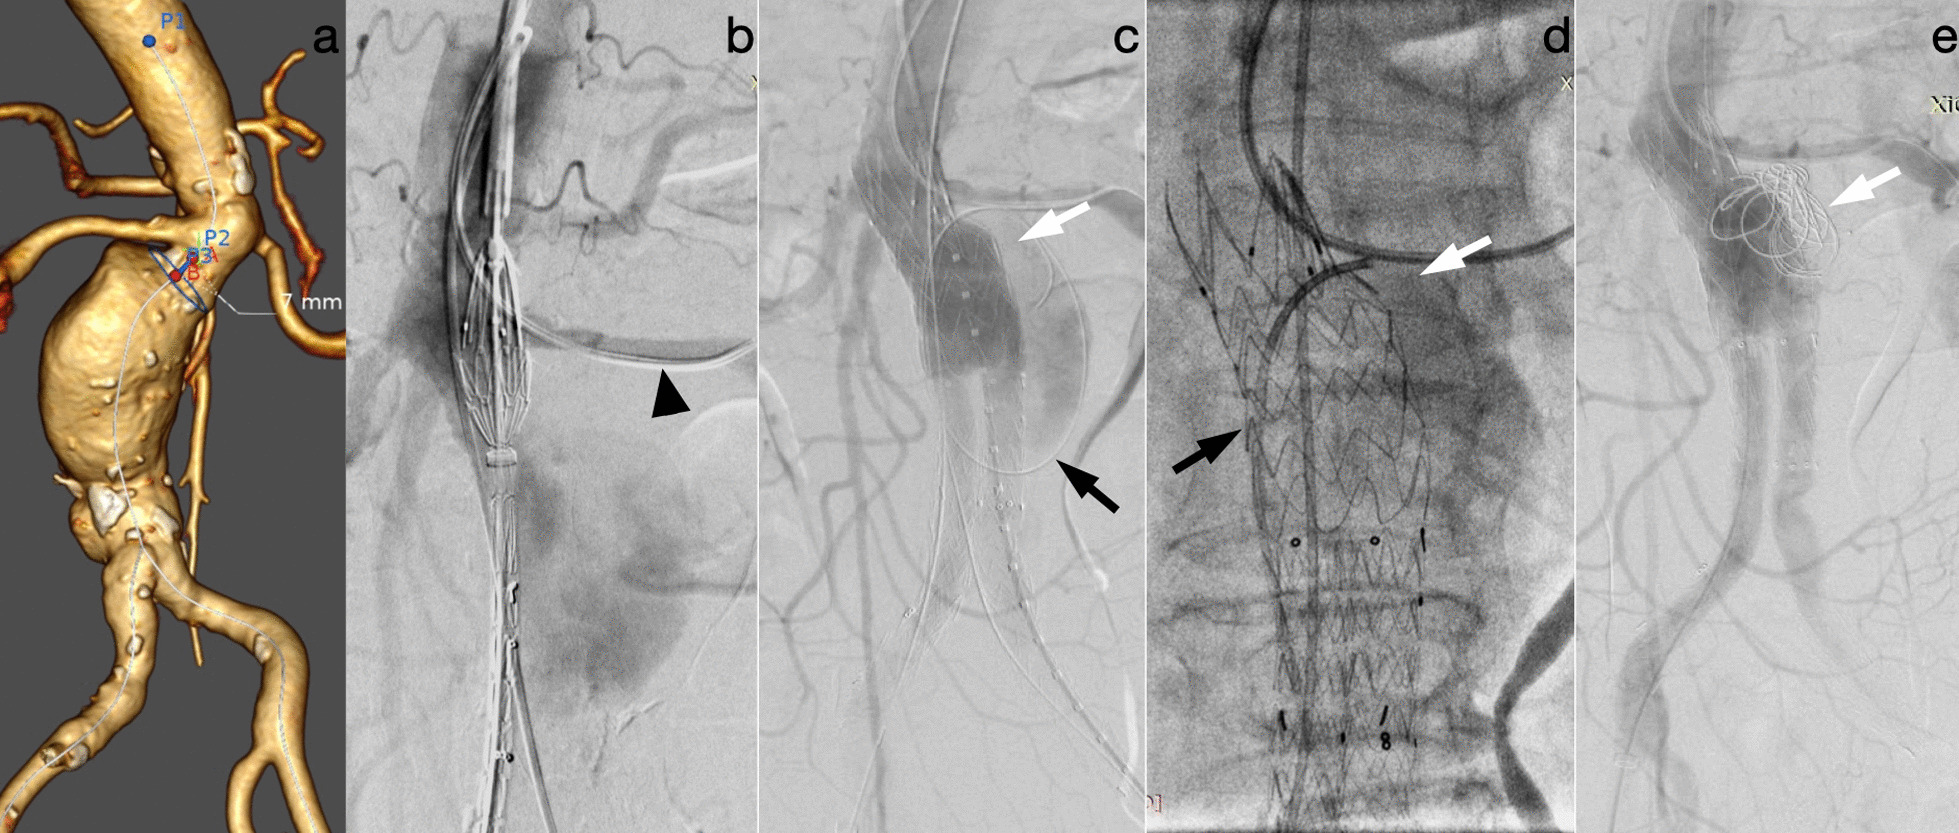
Images of a 77-year-old man who underwent EVAR for the treatment of an AAA with a hostile neck anatomy (aortic neck length, 7 mm). a Three-dimensional reconstruction of CTA demonstrating the short neck length of the aneurysm. b A 5 F catheter (arrowhead) was inserted into the left renal artery via brachial artery access to avoid unintentionally covering the left renal artery. c A 0.035-inch wire was preloaded into the sac (black arrow), and after endograft implantation and balloon moulding, angiography revealed a type Ia endoleak (white arrow). d A 5 F catheter (black arrow) was inserted via the preloaded wire into the sac neck, and saccography confirmed the catheter's position (white arrow). e The coils (white arrow) were delivered via a catheter to the site of the sac neck, and the final angiography confirmed resolution of the endoleak
